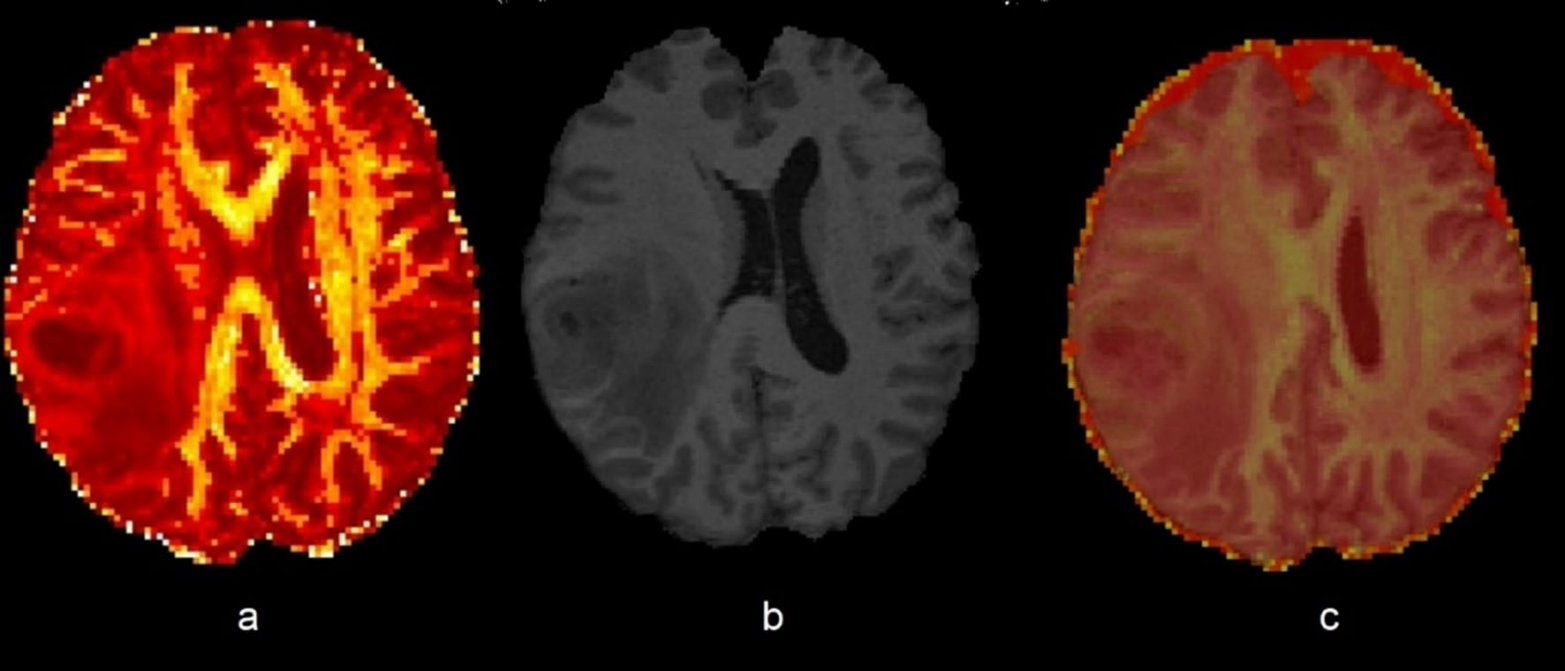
a FA-map, high FA values are yellow, low values are red, b processed T1 MRI , c fusion of MPRAGE T1 and FA-map
